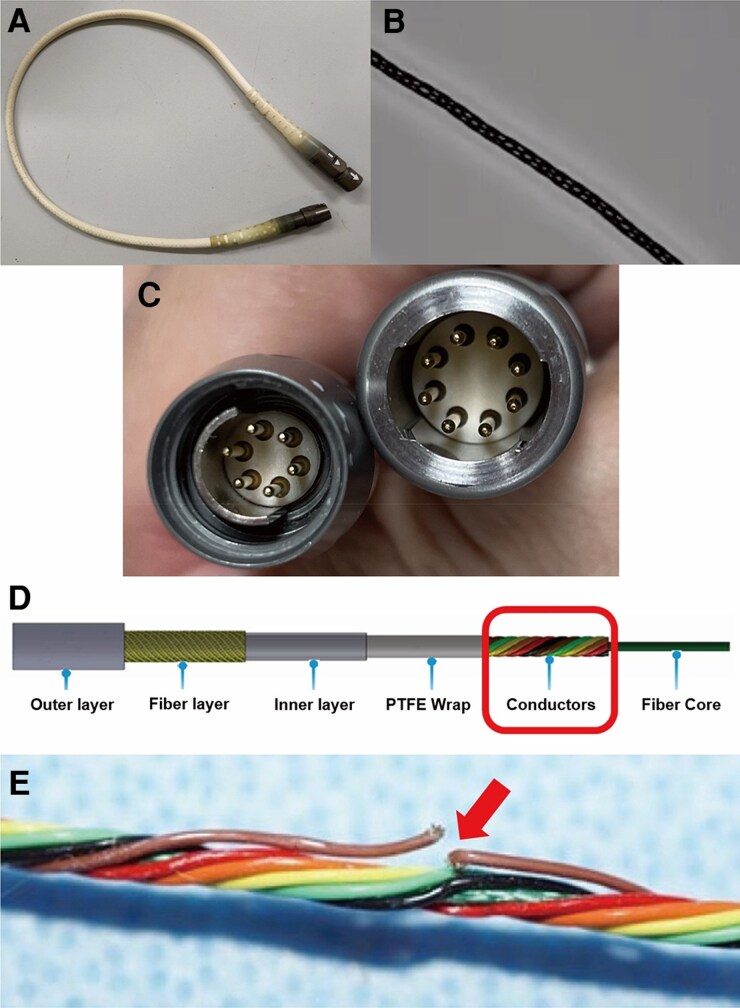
Modular driveline cable in a patient with HeartMate 3. (*A*) Outer layer of the modular cable. (*B*) Modular cable under radiographic imaging. (*C*) Connector between the modular and pump cables. (*D*) Driveline structure (images provided by Abbott Laboratories). PTFE, polytetrafluoroethylene. (*E*, arrow) Internal conductor damage in the modular cable (images provided by Abbott Laboratories).
